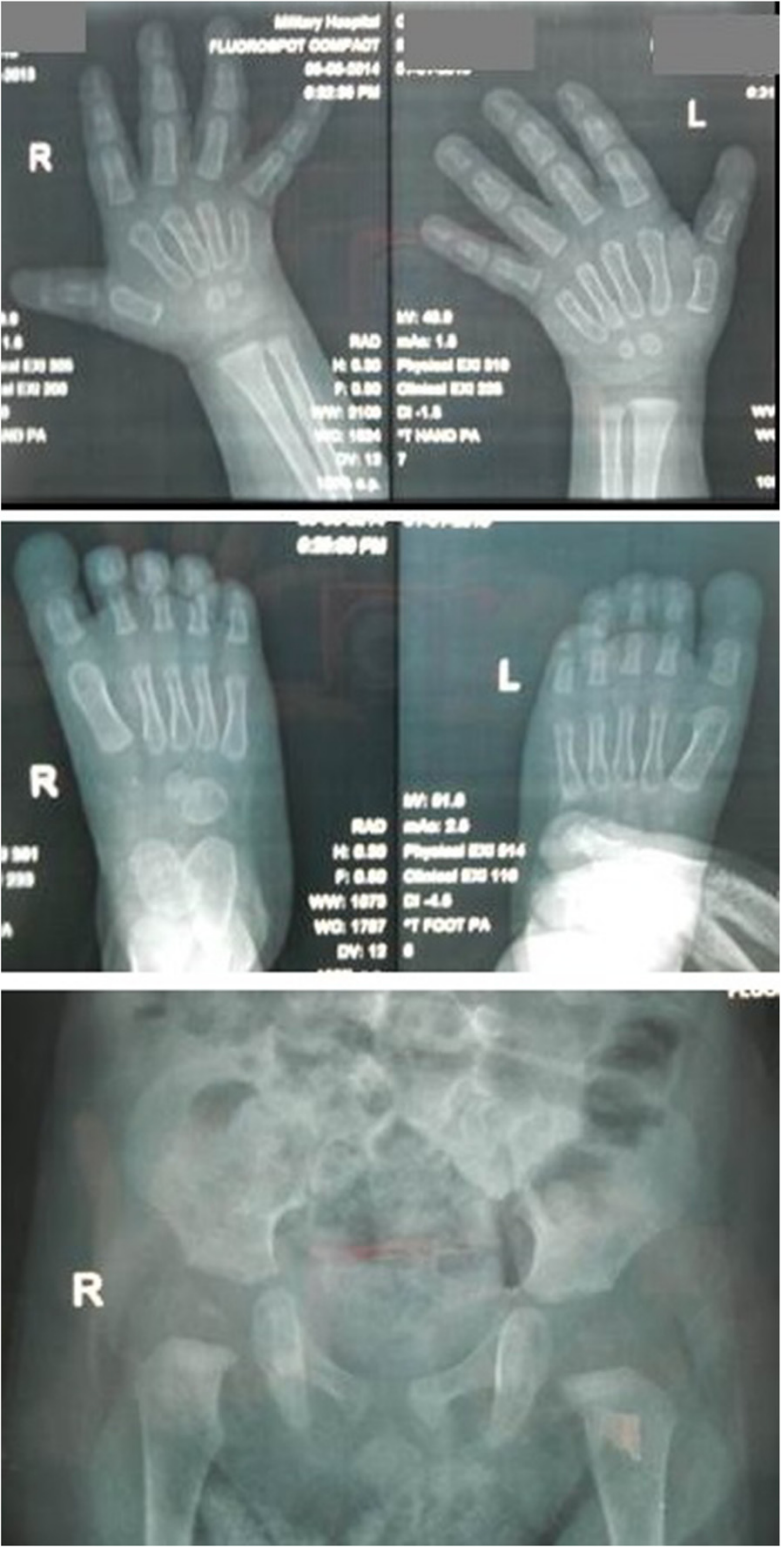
X-ray films of the patient. Note the absent distal phalanges of the thumbs and great toes, and the very small femoral epiphyses at the age of 15 months
